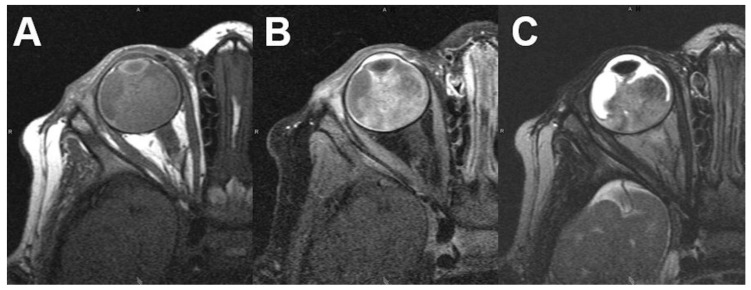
Retinoblastoma*. MRI of a 16-months-old male patient with right-sided retinoblastoma. T1-weighted images show a slightly hyperintense tumor (A) with diffuse contrast enhancement after contrast administration (B). On T2-weighted images the tumor presents with a hypointense signal compared to the vitreous body (C).
